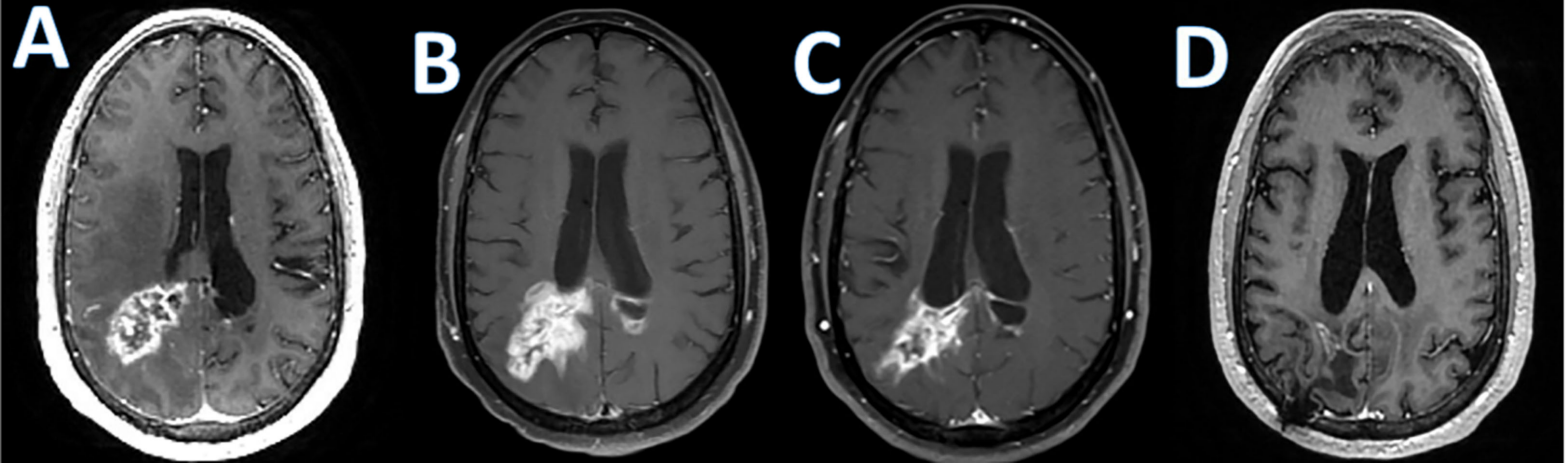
Checkpoint inhibitor associated pseudoprogression. T1 post-contrast enhanced MRI images in a patient with refractory primary central nervous system lymphoma treated with Pembrolizumab; (A); baseline, (B); increase in abnormal enhancement consistent with pseudo progression 9 weeks after starting pembrolizumab, (C); minor response at 7 months; (D); near complete response at 10 months.
